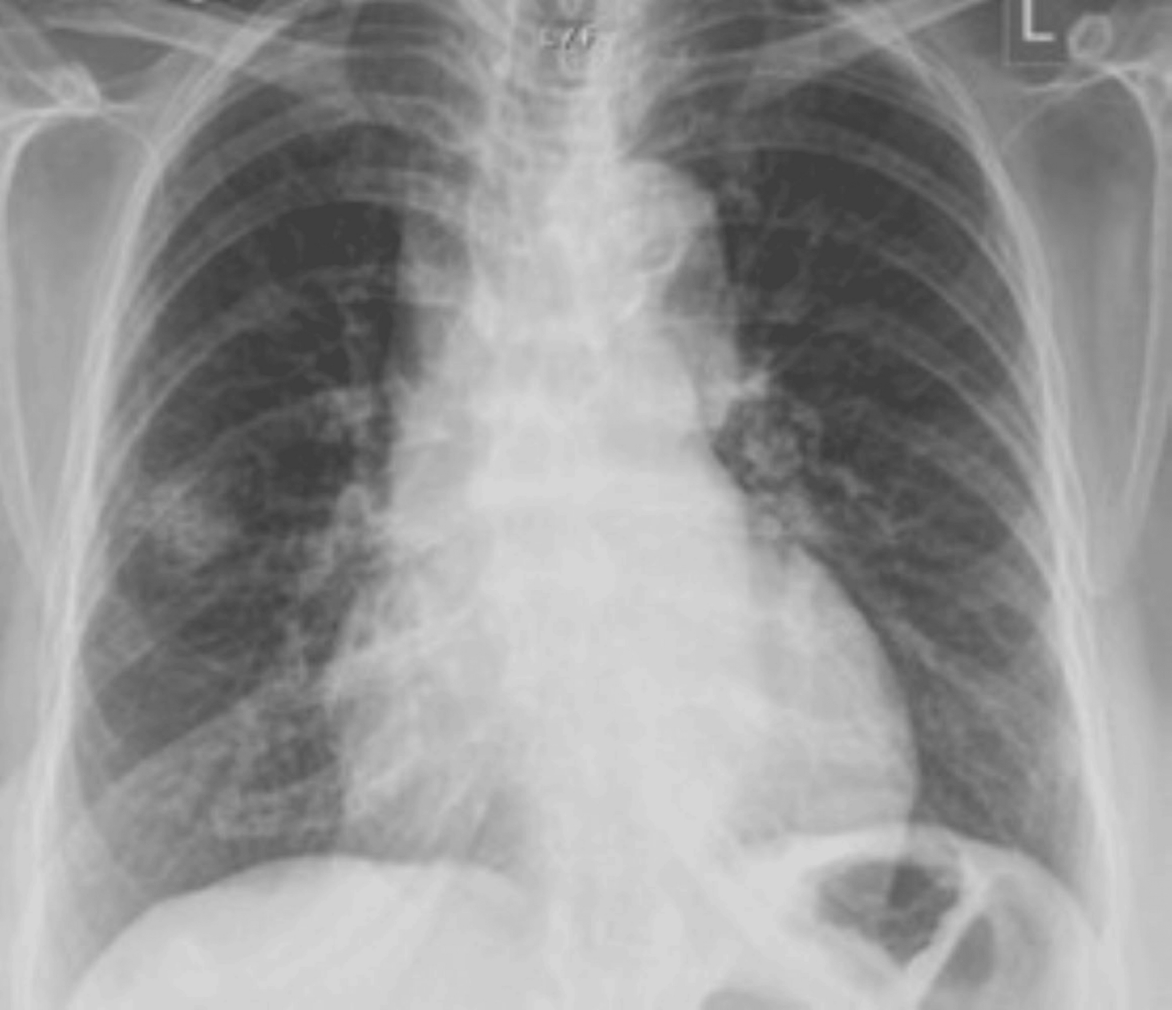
Chest X-ray of Case 2. A 2.2 x 1.3 cm focus of irregular opacification is seen within the right mid-zone.
